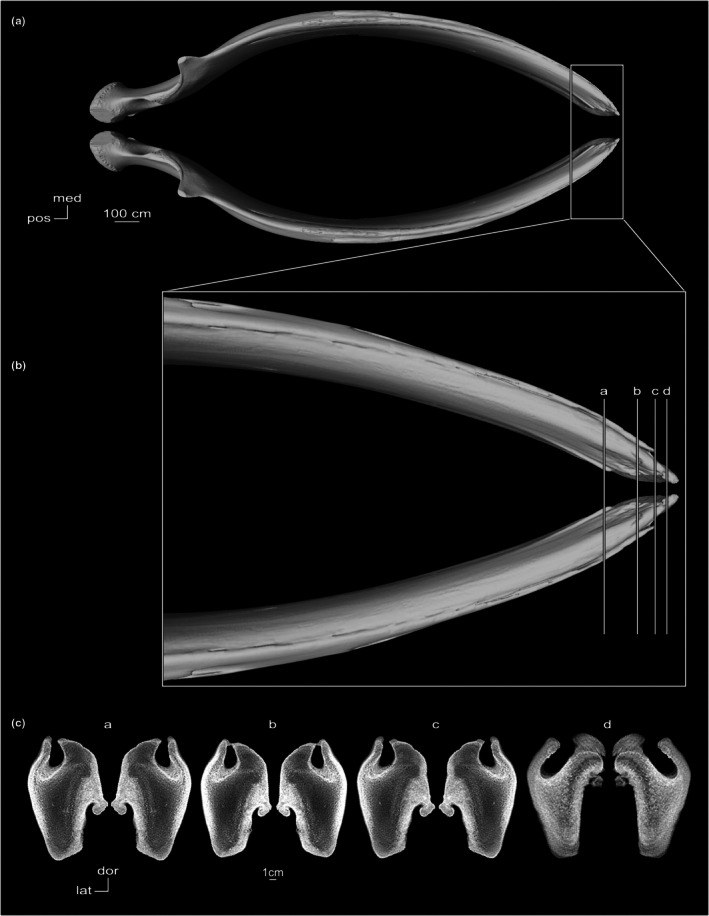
Left mandible of *Megaptera novaeangliae* (DFO 2408), reflected. (a) Dorsal view. (b) Close‐up of the symphysis in dorsal view showing the level of CT cross‐sectional slices (a–d). (c) CT coronal cross‐sectional slices (a–d).
